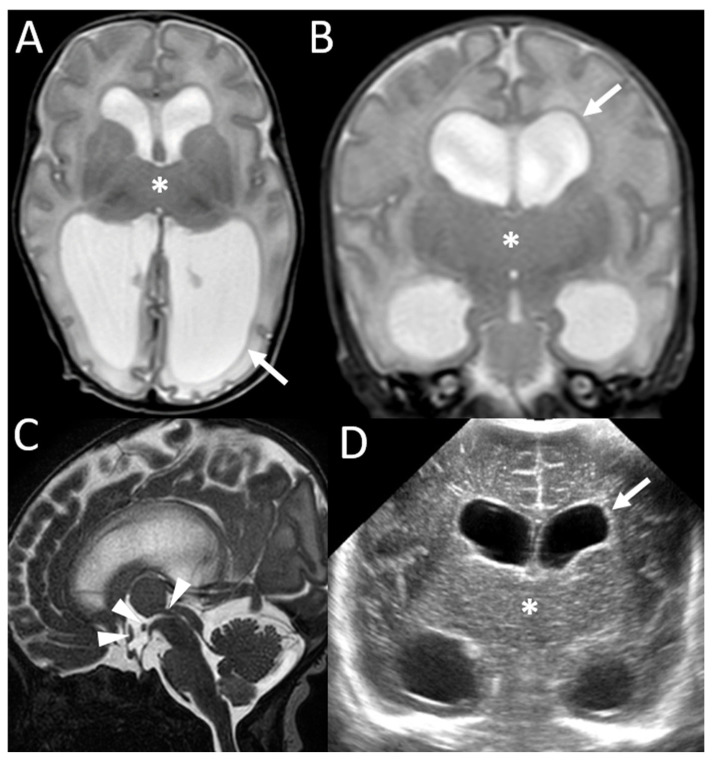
Postnatal MR image ((A) axial, (B) coronal, (C) sagittal T2 weighted images) at 21 days and transfontanellar US image at 1 day (D) confirmed progressive obstructive ventriculomegaly (arrows) upstream from an atretic third ventricle with an aspect of fusion of the thalami (*). Other parenchymal bridges were visible, crossing the midline in the third ventricle and the proximal aqueduct of Sylvius (arrowheads in C on mid-sagittal T2 drive images).
